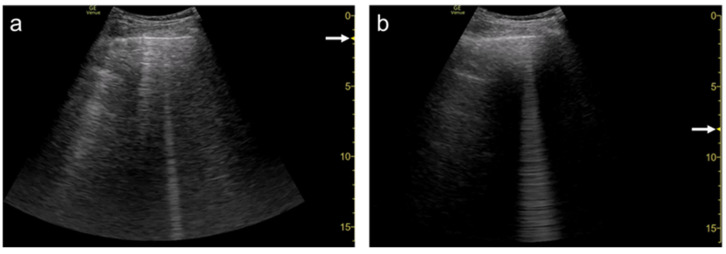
Ultrasound images obtained by Venue scanner (GE Healthcare) with a curvilinear transducer. The B-line becomes wider as the single focal point (arrow) is shifted from the same level as the pleural line (a) to a deeper level (b).
